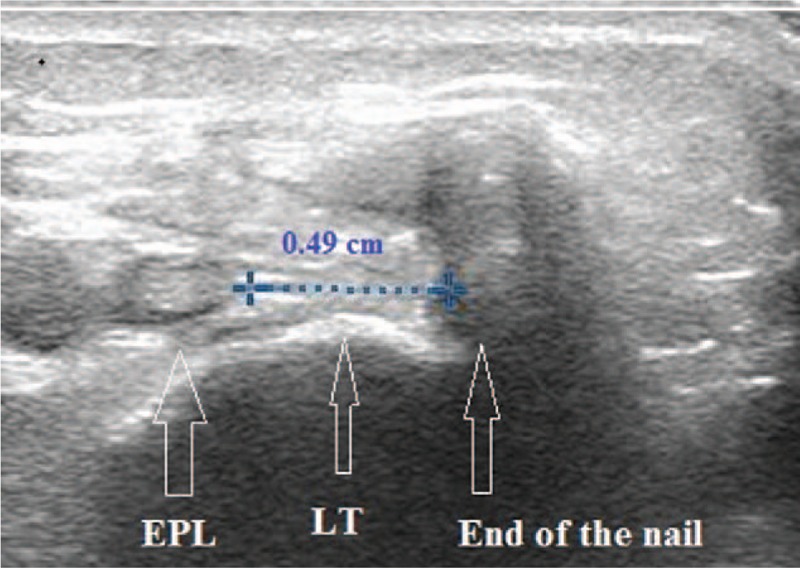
Measurement of the distance of the nail and the tendon in transverse sonographic view. LT = Lister's tubercule, EPL = extensor pollicis longus tendon.
